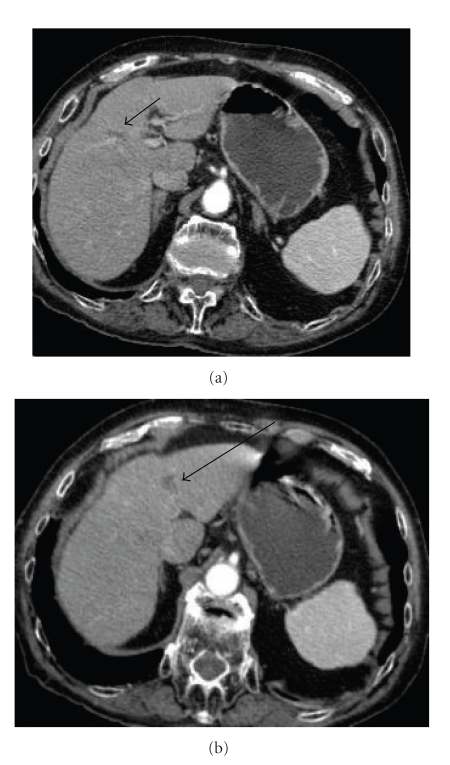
Latest CT scan, 12 years after the initial RF procedure; both lesions (arrows) are hardly visualized and might be misdiagnosed as cystic formations in the liver.
